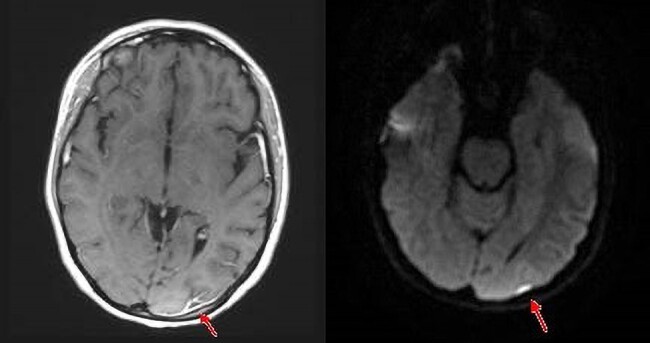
Magnetic resonance imaging brain axial T1-weighted post-contrast (left) shows a small fluid collection with an enhancing membrane overlying the left occipital lobe. Diffusion-weighted imaging (right) shows the collection returns high signal due to restricted water diffusion that is characteristic of pus.
